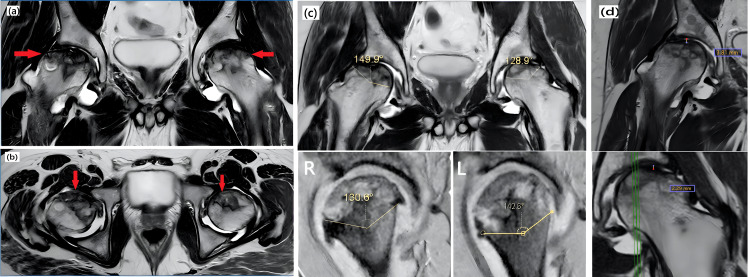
(a) T2W coronal image shows a geographic area of mixed signal intensity with a double line sign bilaterally, predominantly in the antero-latero-superior quadrant extending into the subcapital metaphysis (arrows). Loss of contour of the femoral head with flattening and collapse is seen on the right side. Mild femoral head flattening is seen on the left side. However, the spherical contour is maintained. (b) T2W axial image shows a geographic area of mixed signal intensity with a double line sign bilaterally, predominantly in the antero-latero-superior quadrant, with subchondral cysts (arrows). (c) Modified Kerboul's angle right: -280.5° (149.9°+130.6°); modified Kerboul's angle left: -271.5° (128.9°+142.6°). Findings suggestive of bilateral AVN of the hip joint consistent with ARCO stage IV on the right side and ARCO stage IIIA on the left side. (d) Right hip showing articular collapse of more than 2 mm (3.81 mm) and left hip articular collapse of 2.29 mm. T2W: T2-weighted, STIR: short TI inversion recovery, °: degree, AVN: avascular necrosis, ARCO: Association Research Circulation Osseous
